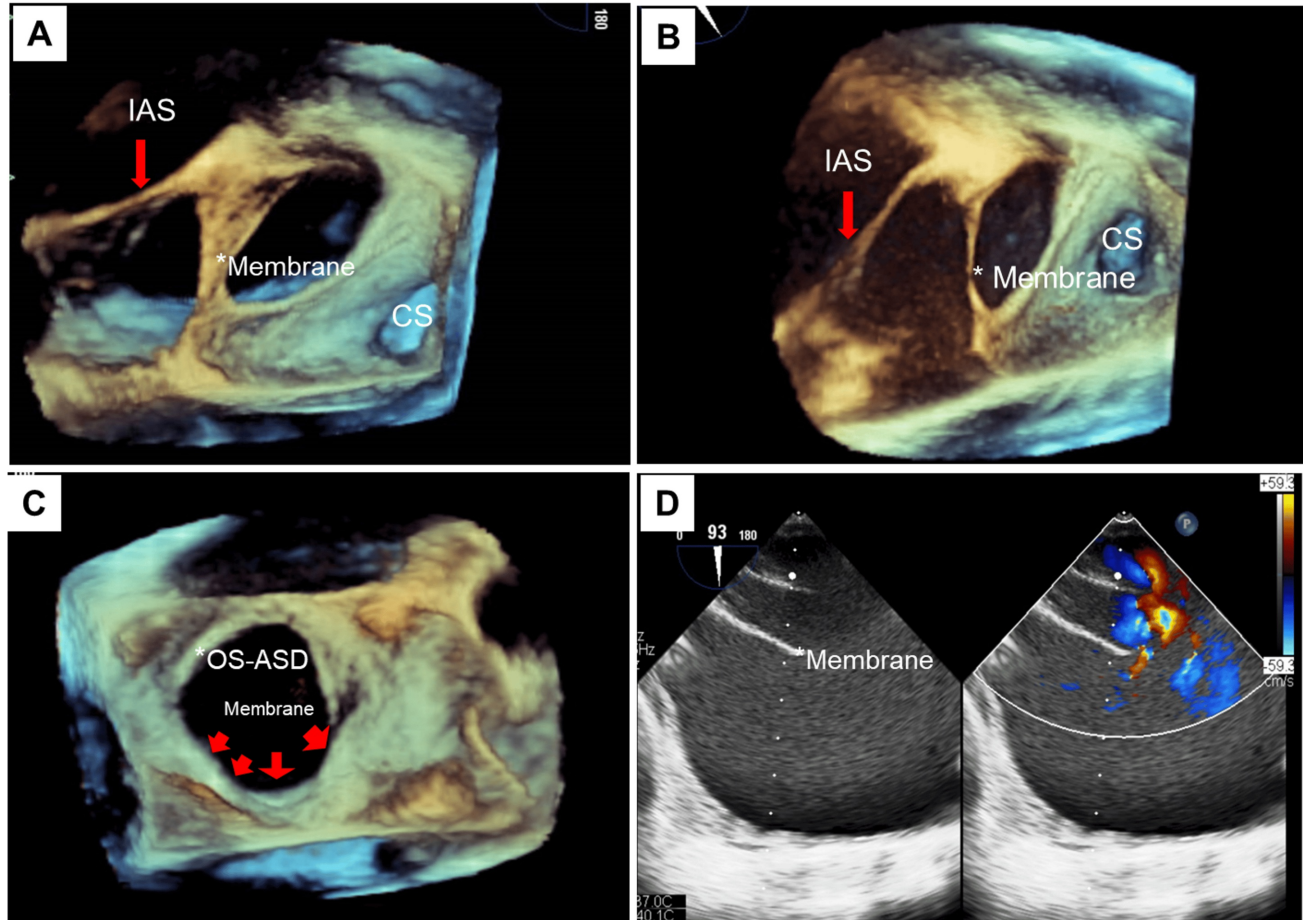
Transesophageal echocardiography images. (A, B) Modified three-dimensional right atrium enface transesophageal echocardiography view images showing interatrial septum (IAS) with ostium secundum atrial septal defect (OS-ASD) with prominent eustachian valve/membrane giving the appearance of a divided right atrium. (C) Three-dimensional transesophageal echocardiography right atrium enface view showing a large OS-ASD. (D) Two-dimensional transesophageal echocardiography bicaval view showing a prominent eustachian valve and the inferior vena cava rim of the atrial septal defect.
